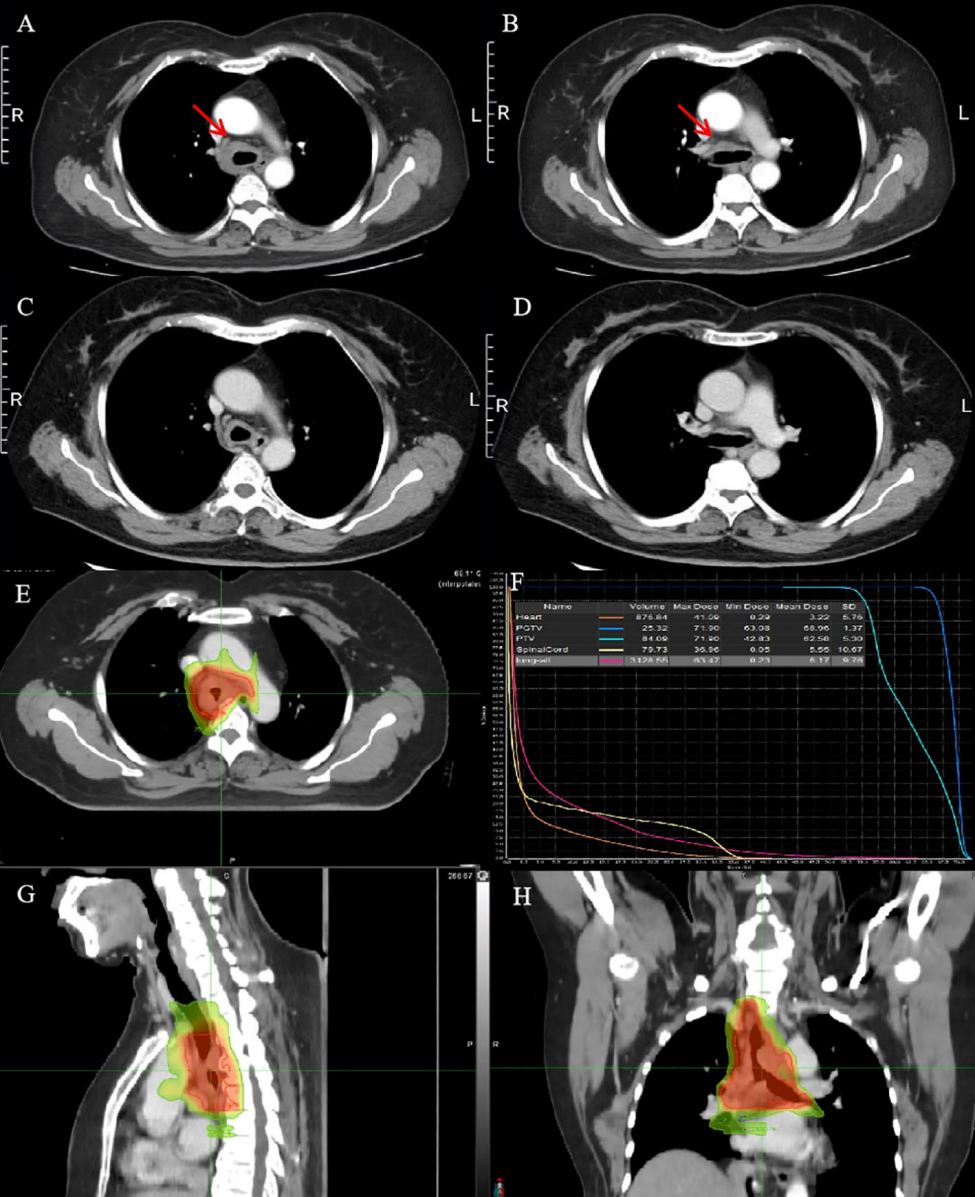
CT images and RT target of case 2 patient. (A, B) Computed tomography (CT) revealed a tracheal lesion. (C, D) The lesion was reduced at 1 month after RT. (E–H) RT target and DVH of case 2 patient; area of red line: 66 Gy; and area of yellow line: 54 Gy.
